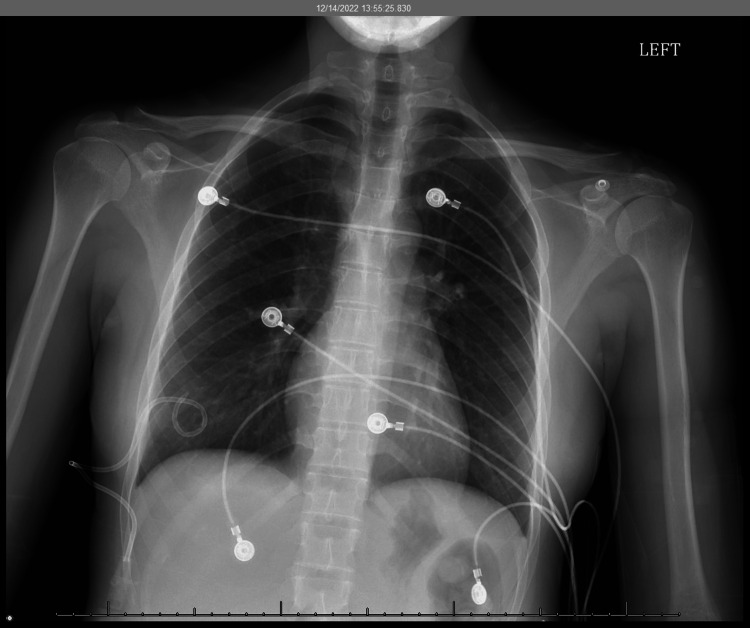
Initial chest x-ray Impression: Right pleural pigtail catheter tip in the right lung base. No pneumothorax and no effusions.
